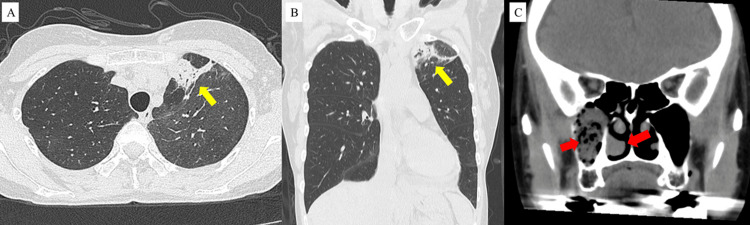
Chest and paranasal sinuses computed tomography Chest computed tomography (CT) shows a consolidation without multiple nodular and cavitary lesions in the left upper lobe (yellow arrow) (A, B). Paranasal sinuses CT shows occupying lesions in the right maxillary sinus and mucosal thickening of the middle turbinate and inferior turbinate on the right side (red arrow) (C)
